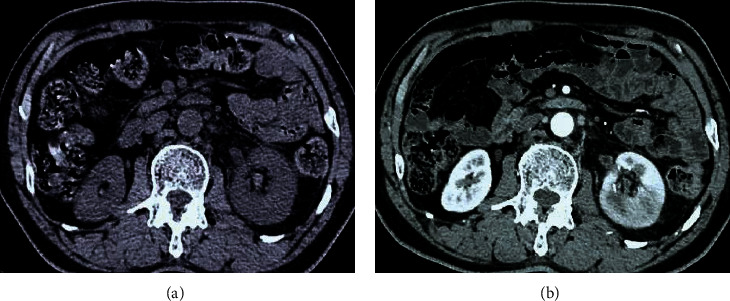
CT image of a patient's kidney (female, 50 years old). The left image was the CT plain scan result, and the right image was the CT enhanced result.
